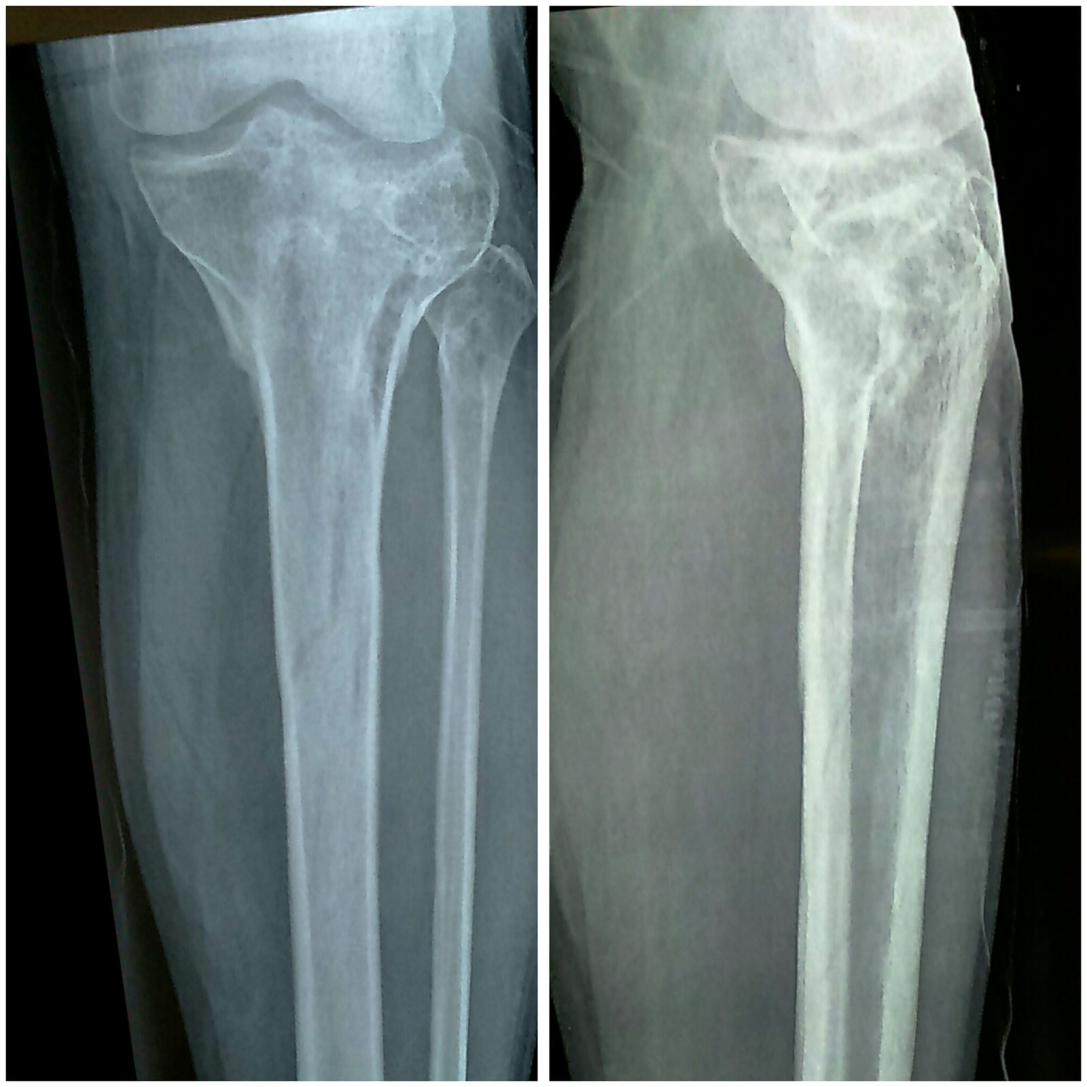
Anteroposterior and lateral X-ray views at eight months of follow-up.
